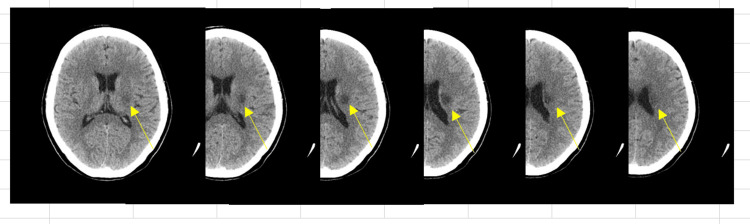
Computed tomography imaging A low-density area from the corona radiata to the basal ganglia was observed.
